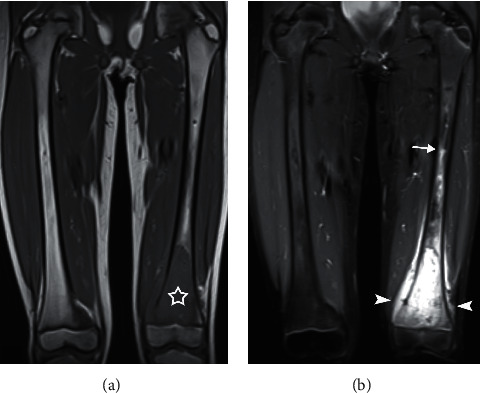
Magnetic resonance imaging (MRI) of the thighs. Coronal T1-weighted (a) and fluid-sensitive (b) large-field-of-view images confirm a marrow replacement process centered within the left distal femoral metaphysis (star) with extraosseous extension (arrowheads) and proximal marked peritumoral bone marrow edema-like signal (arrow).
